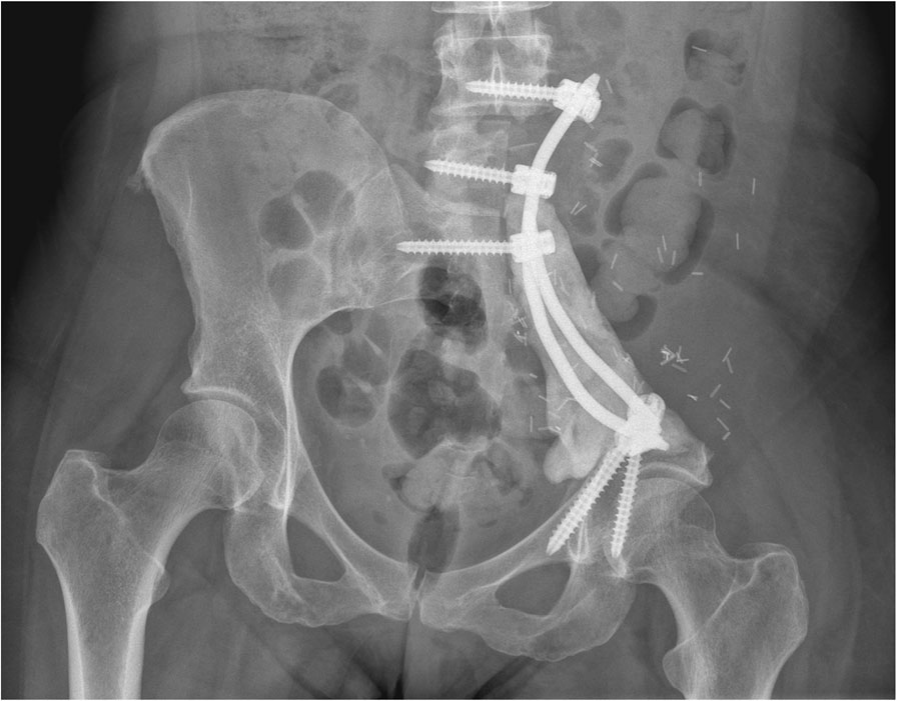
Plain radiograph of a pelvis a.p. after type 1c resection including partial resection of the fifth lumbar vertebrae and reconstruction with a polyaxial screw-rod-system augmented by a bone cement sheath
